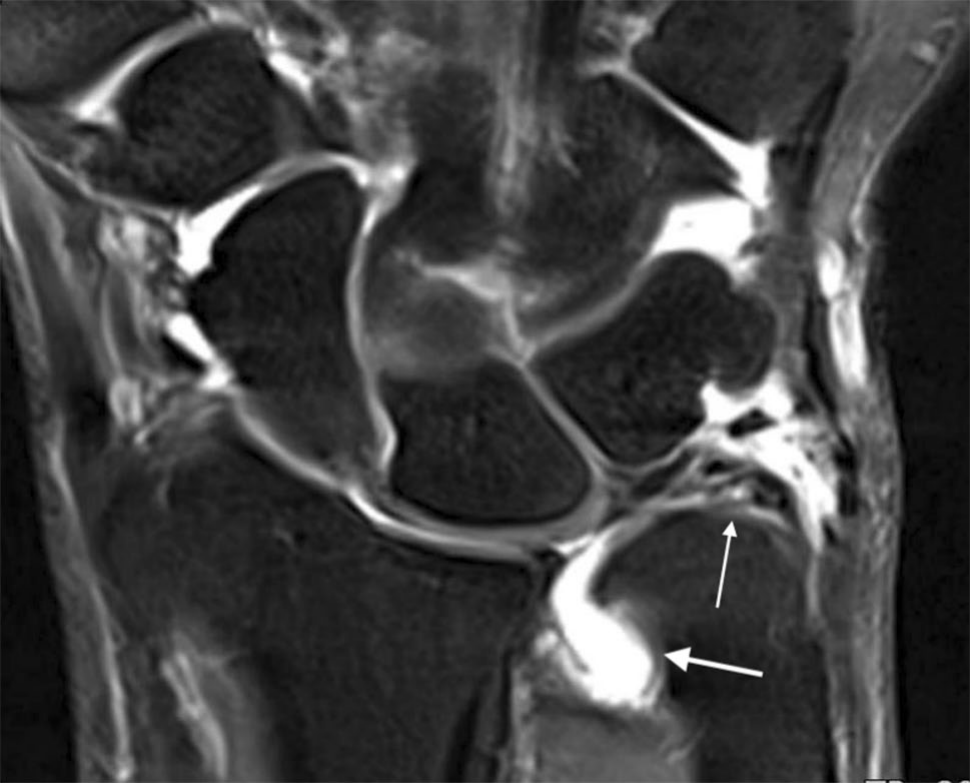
Coronal T2-weighted wrist MRA showing a TFCC tear (thin arrow) with contrast leakage (thick arrow)
